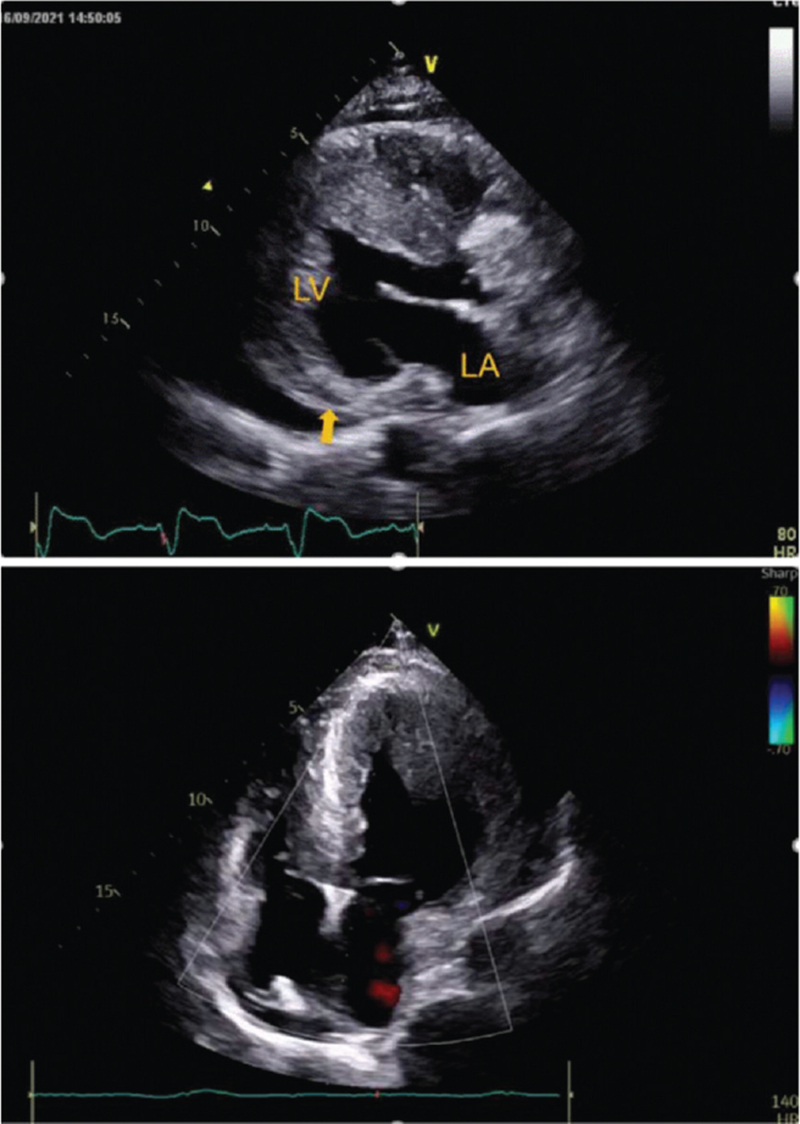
Echocardiogram conducted at our hospital: hypertrophy, ejection fraction 40%, pericardial effusion.
